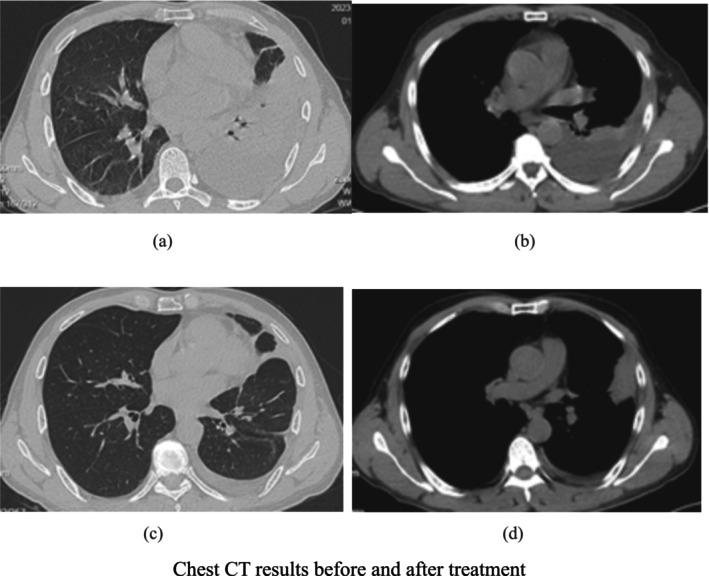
Chest CT results before and after treatment. (a,b) Emergency chest CT on October 22, 2023. (c,d) Chest CT from the Department of Respiratory and Critical Care Medicine on November 2, 2023.
